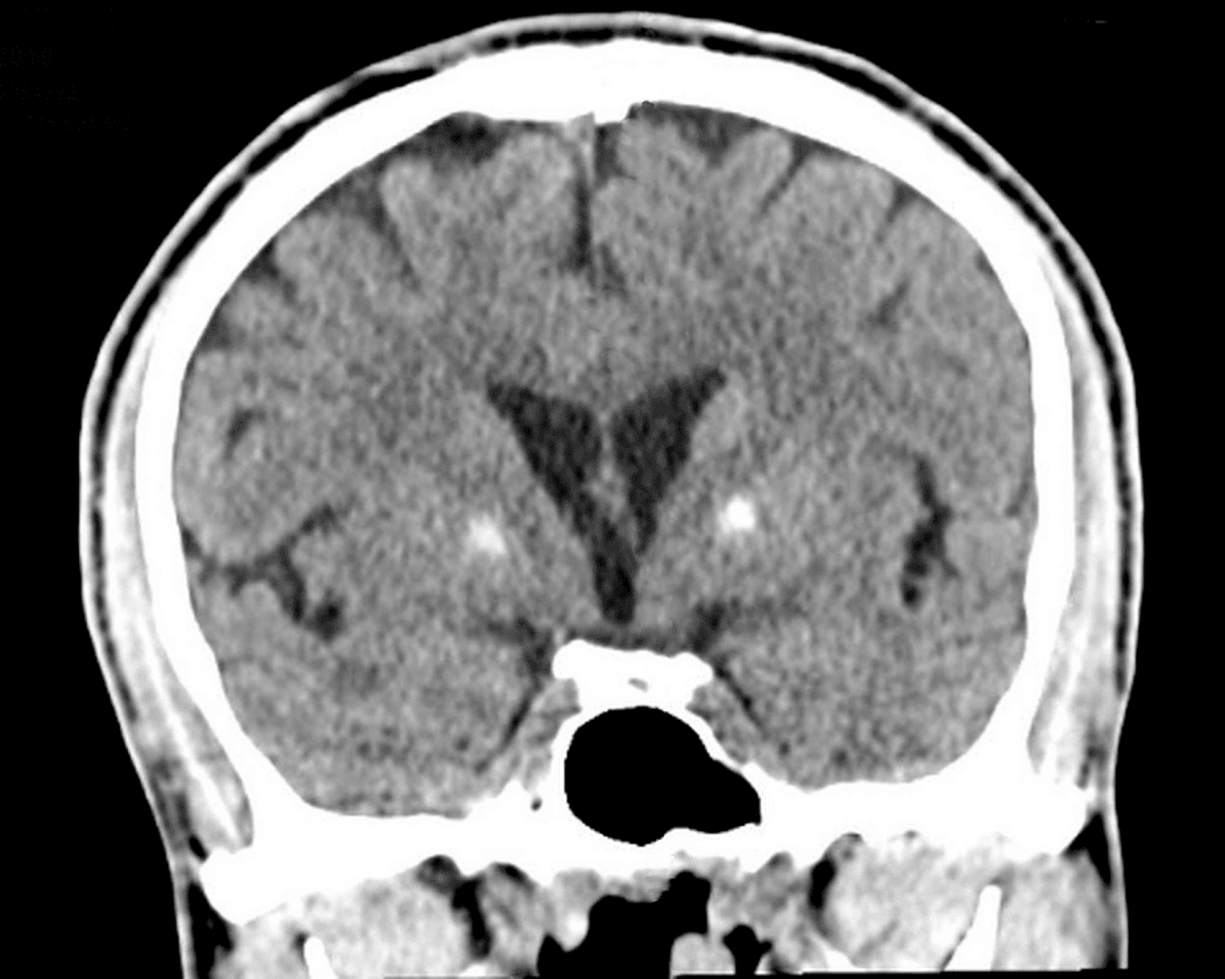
A coronal plane CT scan of the patient's brain showing calcification limited to the anterior region of the globus pallidus externus.
